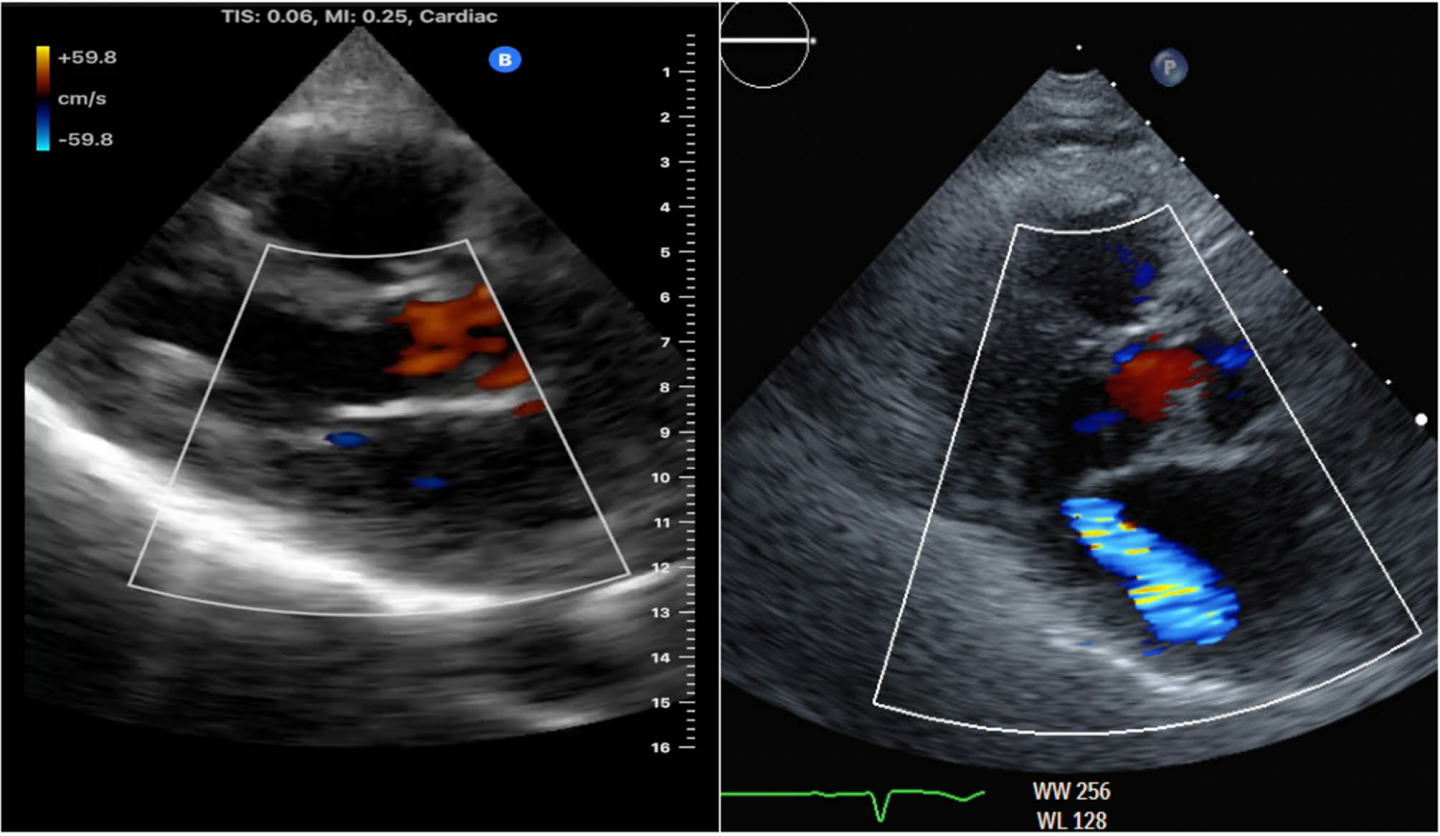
Comparison of a significant MR imaged on the Butterfly IQ+ (left) vs. cart-based Echocardiography (right)
